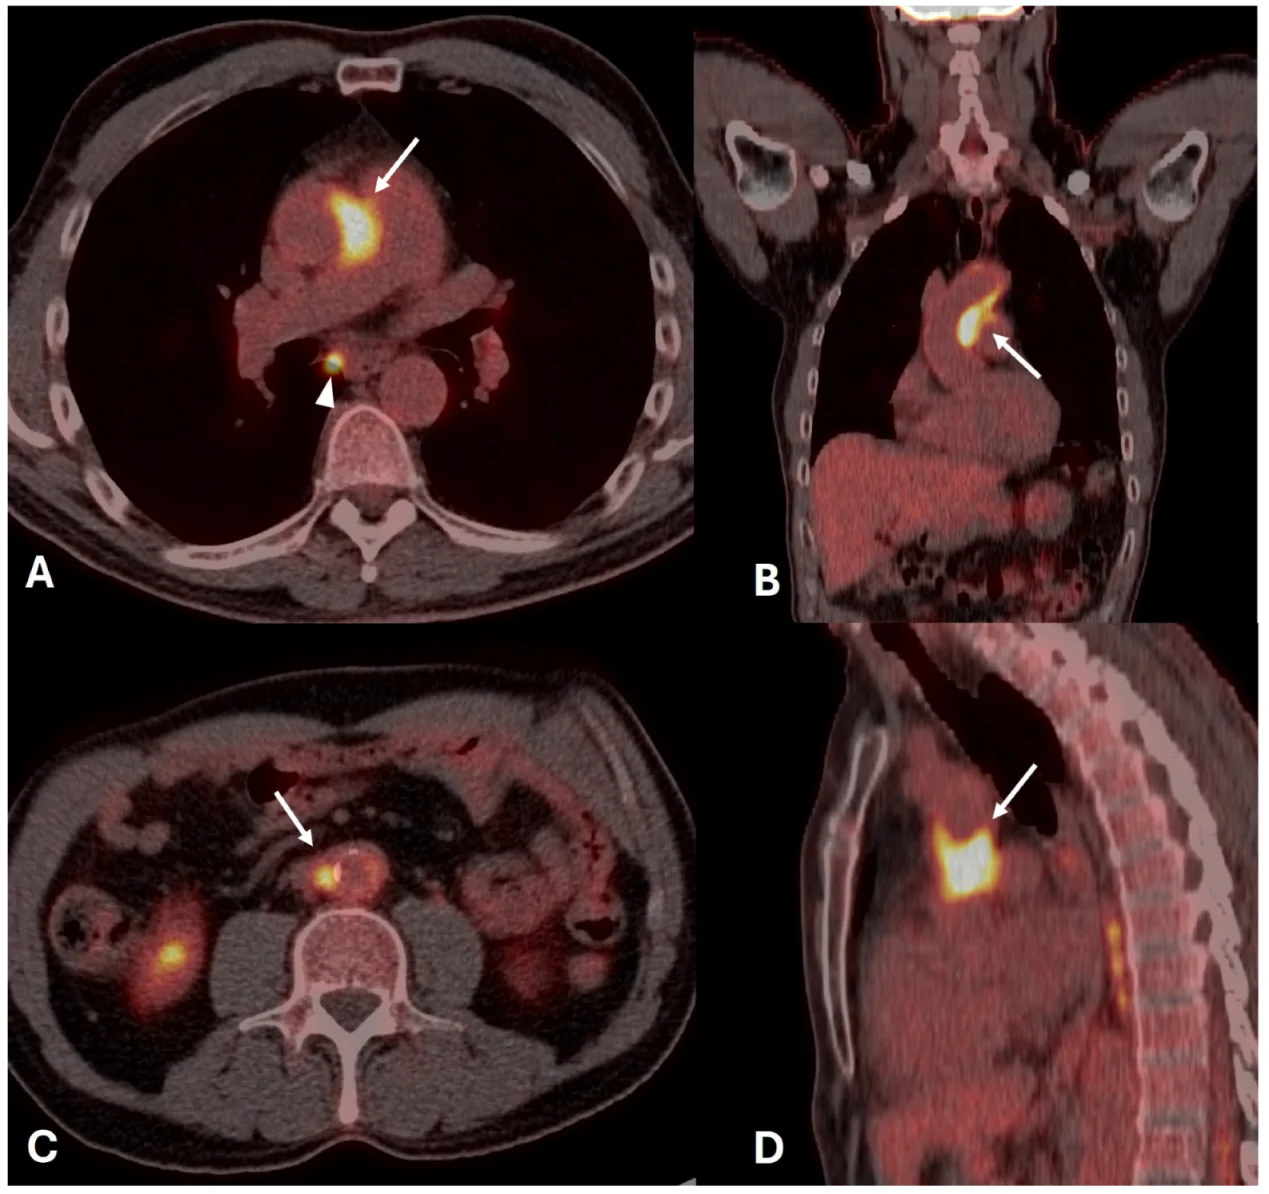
An example of known IgG4 disease evaluated for persistent elevated inflammatory markers. Axial (A,C), coronal (B) and sagittal (D) images of F-18 FDG PET/CT showing tracer avid mural thickening involving the ascending aorta, proximal aortic arch, and upper abdominal aorta (arrows) indicating active vasculitis. There is a subcentimeter tracer avid mediastinal level 7 lymph node (arrowhead) which is likely inflammatory.
